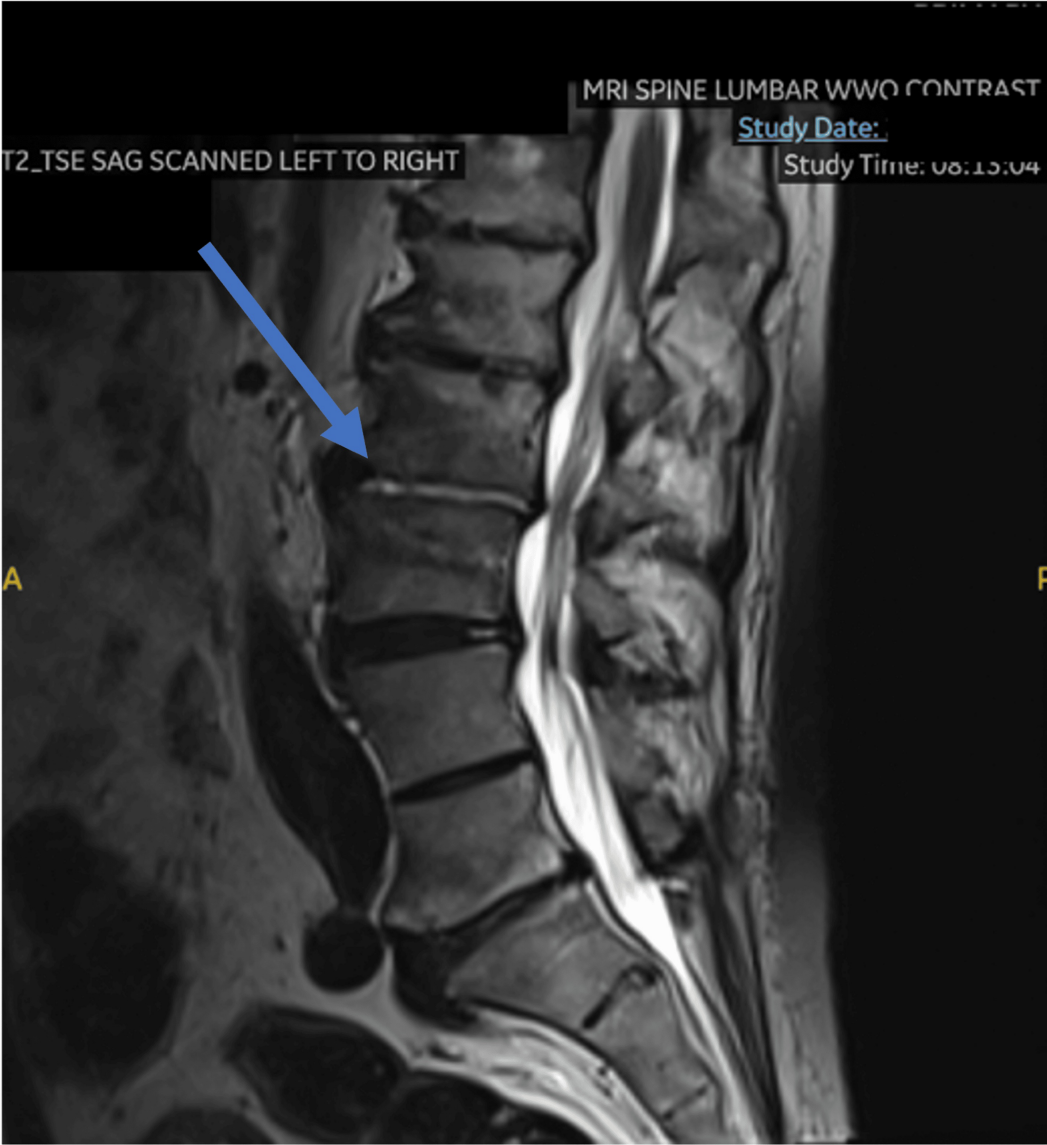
MRI of lumbar spine showing L2-3 osteomyelitis/discitis
